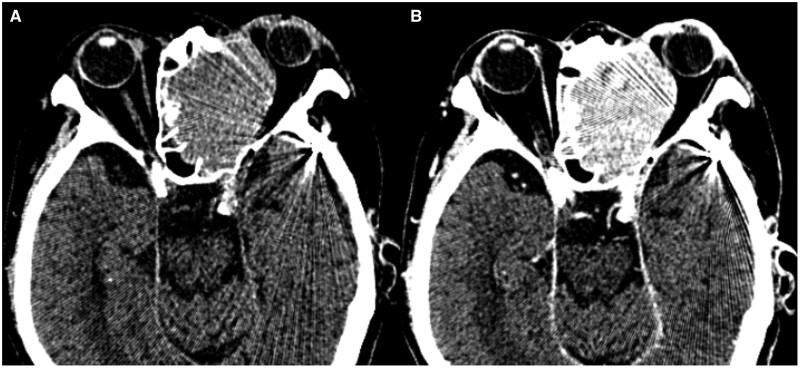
(A, B) Follow-up CT after biopsy on the day 14 of admission. Non-contrast CT shows a homogeneous high-density mass in the left ethmoid sinus extending to the left orbit (A). Contrast-enhanced CT shows homogenous enhancement in the tumour (B).
